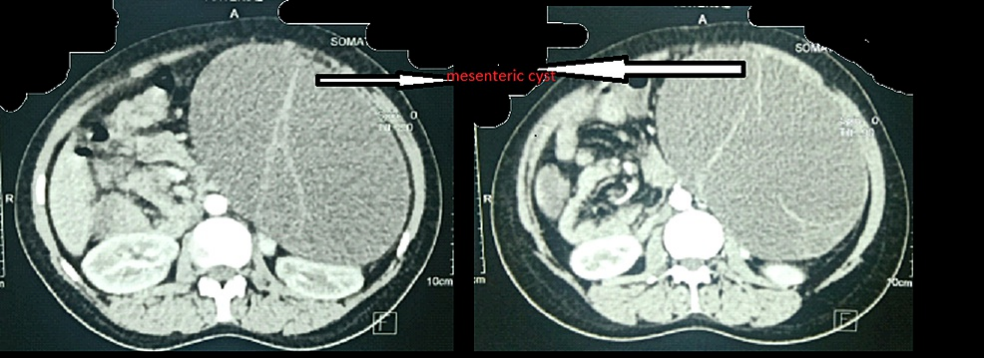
CT image of the abdomen The CT scan reveals a large, well defined multiloculated thin-walled cystic lesion with thin incomplete enhancing septae.
